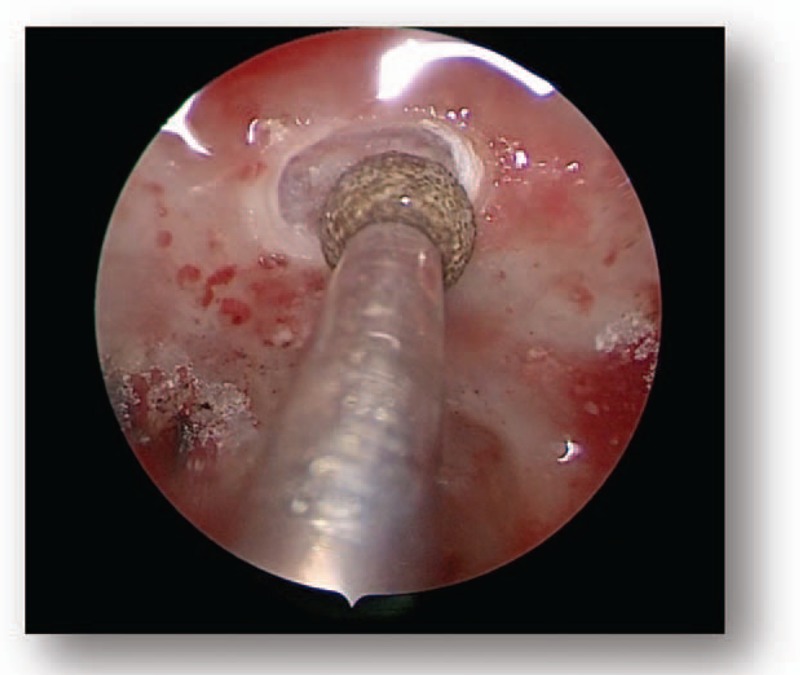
Preoperative MRI (C). MRI = magnetic resonance imaging.
